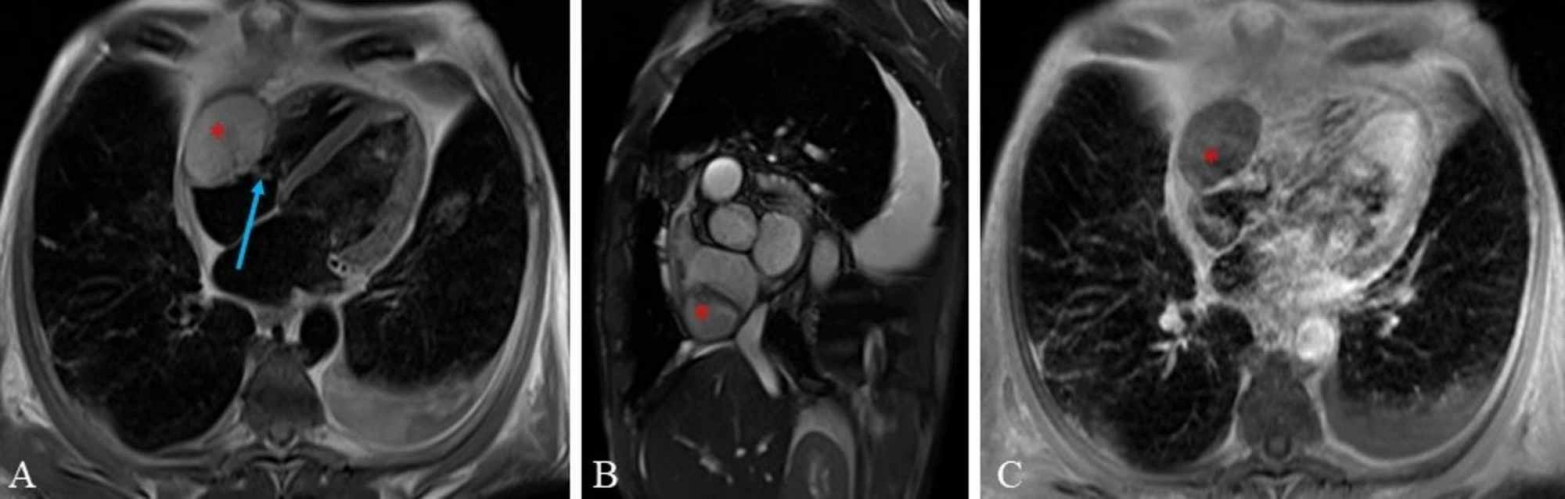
Cardiac magnetic resonance imaging (A) Four chamber view T2 dark blood magnetic resonance imaging (MRI); (B) short axis view cine steady-state free precession MRI; and (C) four-chamber view. T1 fat saturated post-gadolinium contrast MRI demonstrates a non-enhancing mass (asterisk) extending between the right atrium and right ventricle through the tricuspid valve. The mass has a friable component in the right atrium that is freely mobile (arrow). This mass was thought to represent a thrombus, with or without superinfection.
